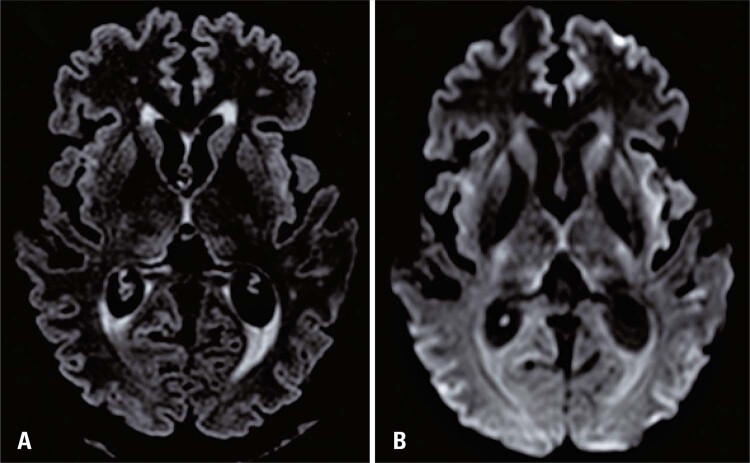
Axial magnetic resonance imaging. (A) FLAIR imaging sequence with hyperintensity involving practically the entire cortical ribbon; (B) Diffusion-weighted imaging sequence with diffusion restriction throughout the cerebral cortex, notably in the bilateral insulae
